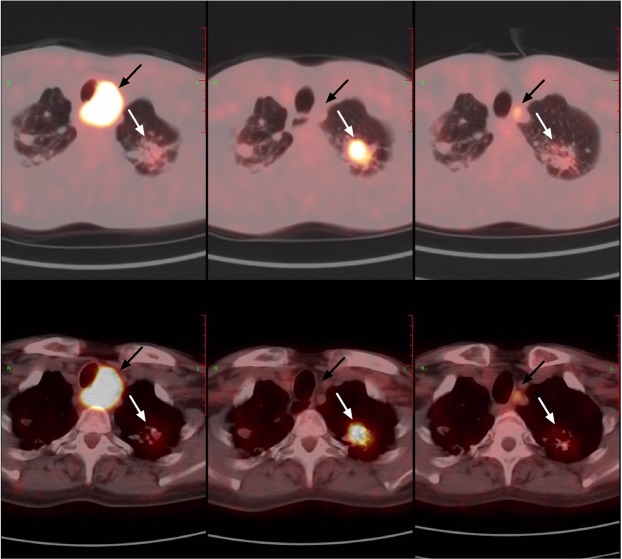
A 60-year-old man with history of lung cancer. Old tuberculosis lesions were seen at the apex of both lungs, and a metastatic lymph node can be seen beside the trachea. After 5 months of chemotherapy, the PET/CT follow-up showed that the volume of old tuberculosis in the left apex pulmonis increased, and the SUVmax increased from 2.6 to 7.5 (white arrow); the paratracheal high metabolism lymph node volume was reduced and metabolism was essentially disappeared (black arrow). After 4 months of anti-tuberculosis treatment, the PET/CT follow-up showed that the metabolism of hypermetabolic tuberculosis decreased, but the volume of metastatic lymph nodes increased slightly and metabolism increased again.
